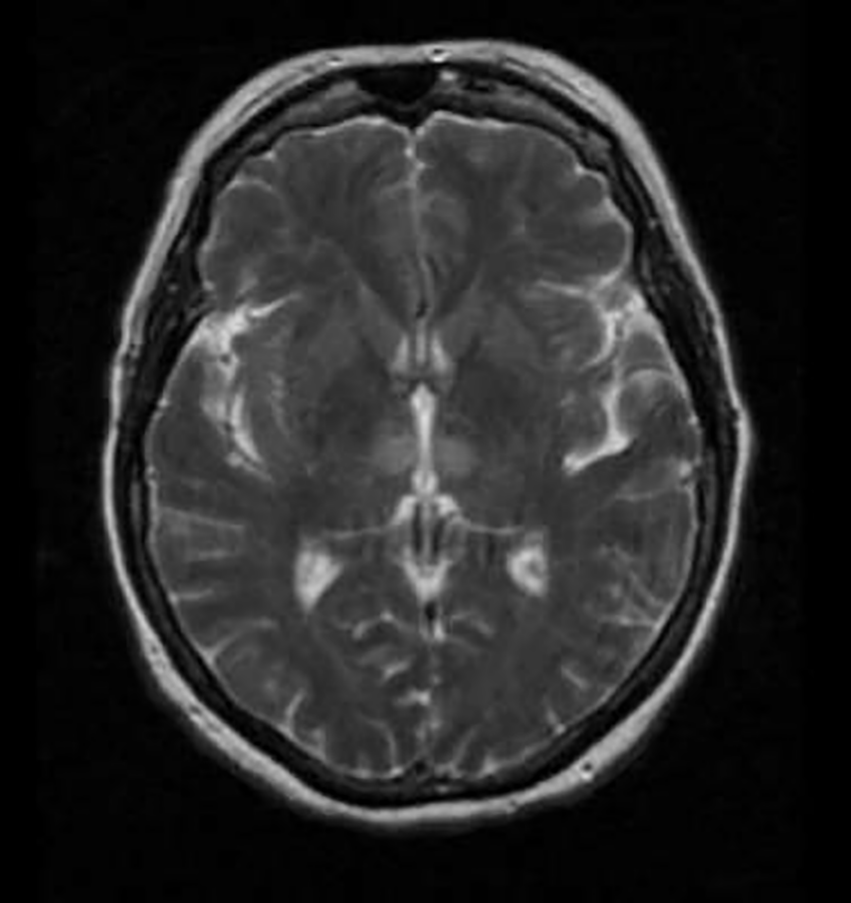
T2/FLAIR signal weighed axial MR demonstrating hyperdensities of bilateral paramedian thalami.
